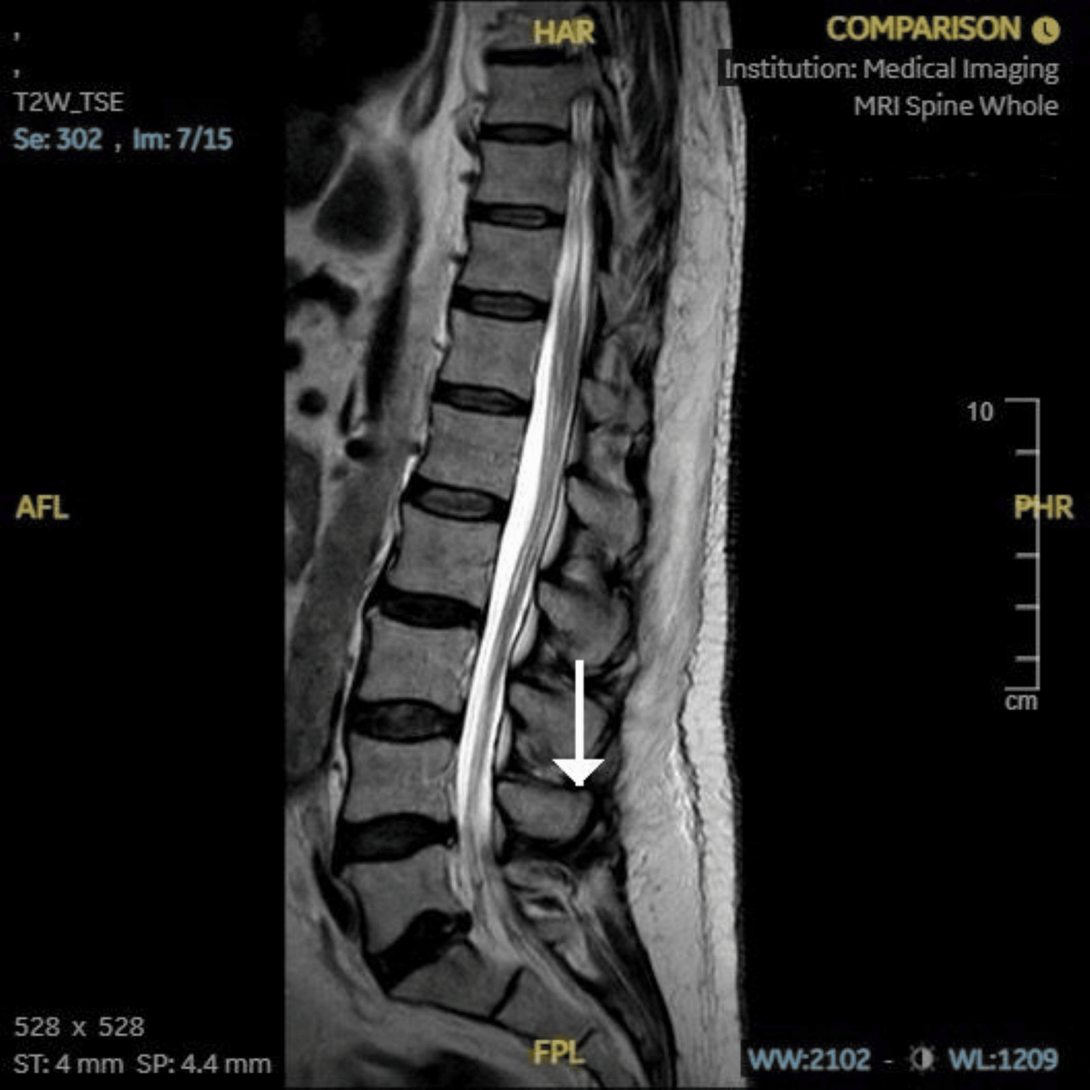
MRI of the lumbar spine (sagittal T2-weighted sequence) demonstrating preserved vertebral alignment and no abnormal signal changes. A white arrow highlights the lumbar spine region of interest.
